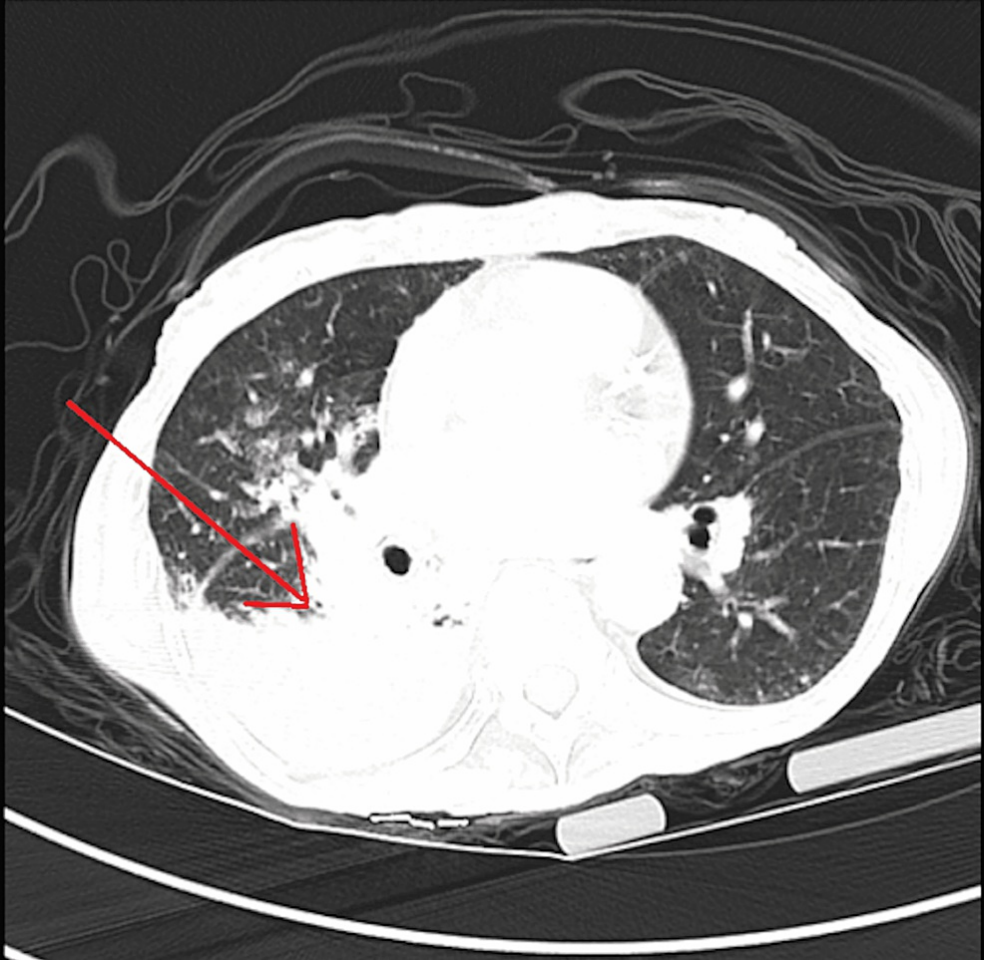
Axial CT scan without contrast identifying bilateral pulmonary infiltrates with a large air-fluid collection (pointed to by the red arrow) within the right lobe
